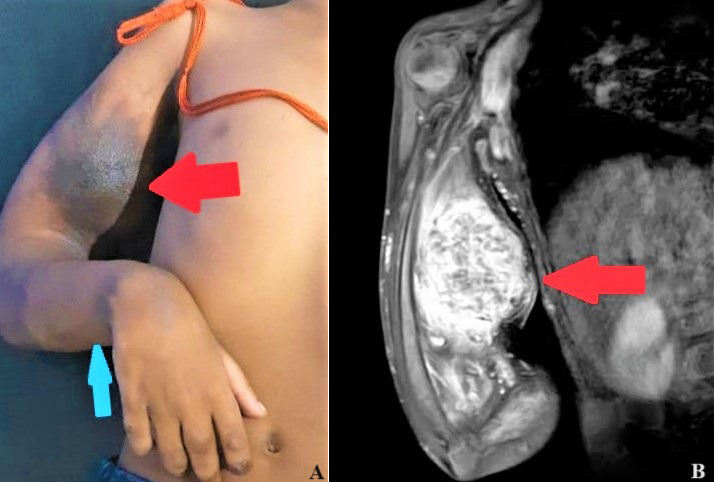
A) Magnetic Resonance Imaging (MRI) findings of bulging and blue-blackish discolouration, red arrows show oval lesion and blue arrow show skin blue colouration at intermuscular compartment of proximal 1/3^rd^ of right forearm; B) MRI findings of arm arteriovenous malformation- red arrows show oval lesion of arteriovenous malformation
